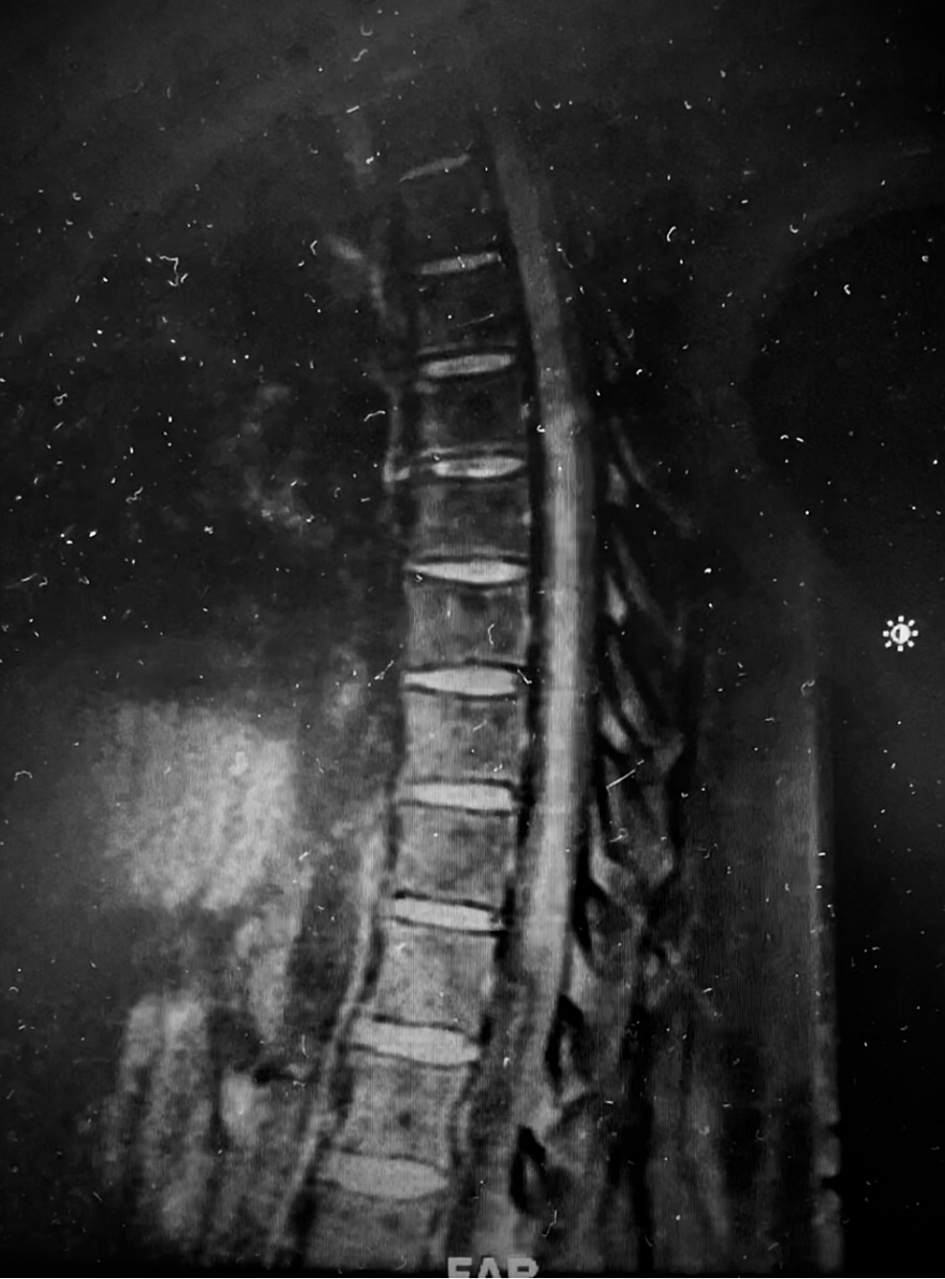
T1-weighted thoracic spine MRI pre-contrast (gadolinium).
